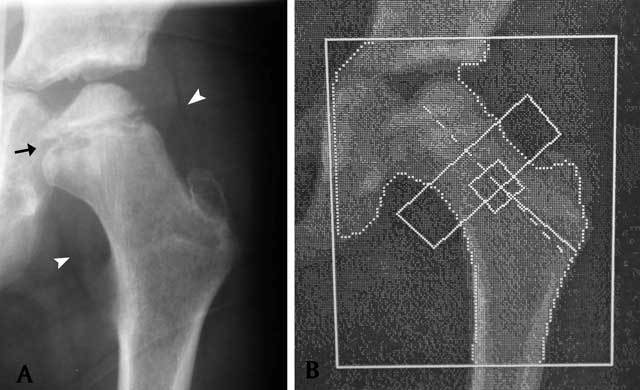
(A) The initial frontal radiograph of the left hip at 8-year age (phase of disease onset) shows early abnormalities including a sclerotic femoral ossification center that is laterally displaced, metaphyseal irregularity (arrow) with cyst formation, and mild soft tissue distortion (arrowheads). (B) DXA scan shows subtle osteosclerosis of the left hip. The BMD value of the total hip is 0.481 g/cm^2^.
